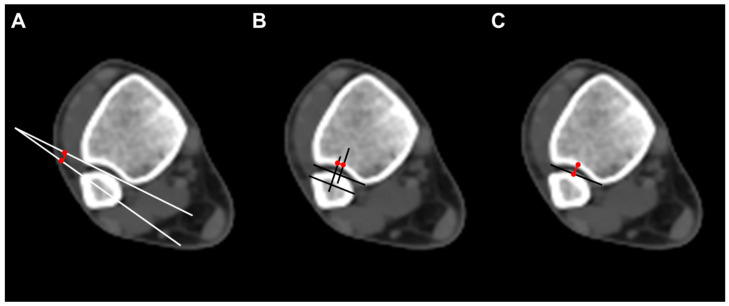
Radiographic measurements. (A) Fibular rotation (angle between a line drawn between the anterior and posterior borders of the incisura and the fibular orientation line); (B) sagittal translation (difference between the midpoint of the incisura length and the midpoint of the fibular length line. The fibular length line was drawn from the most anterior point of the fibula, parallel to the incisura length); (C) incisura depth (distance from the tibial cortex at the midpoint of the incisura to the line that joins the most anterior point with the most posterior point of the incisura).
